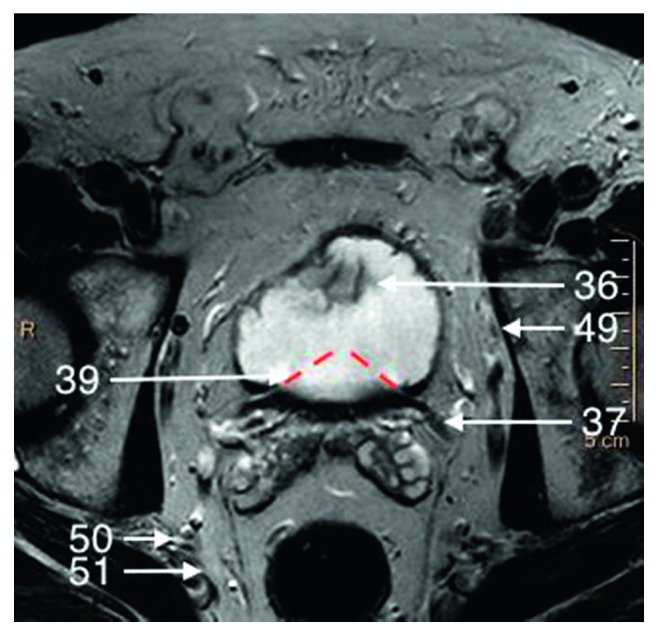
T2-weighted axial image of a basal part of the prostate gland.
